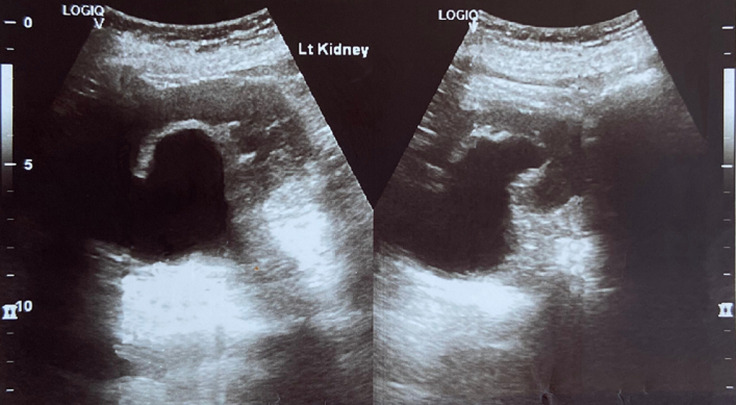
preoperative ultrasound imaging of the right kidney
